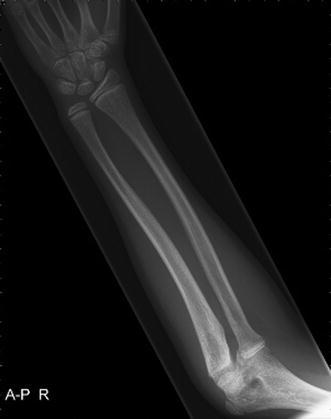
AP radiograph of the right forearm. A mild bowing of the ulna can be observed
